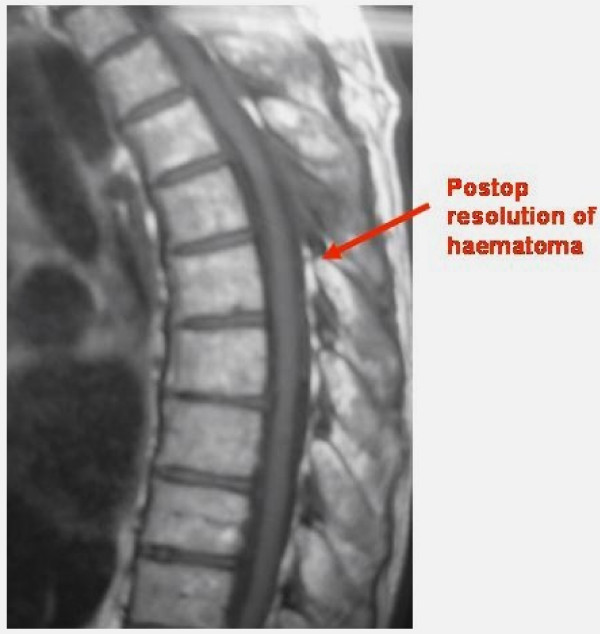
Six month post-op MRI- T1 Sagittal showing resolution of haematoma.
